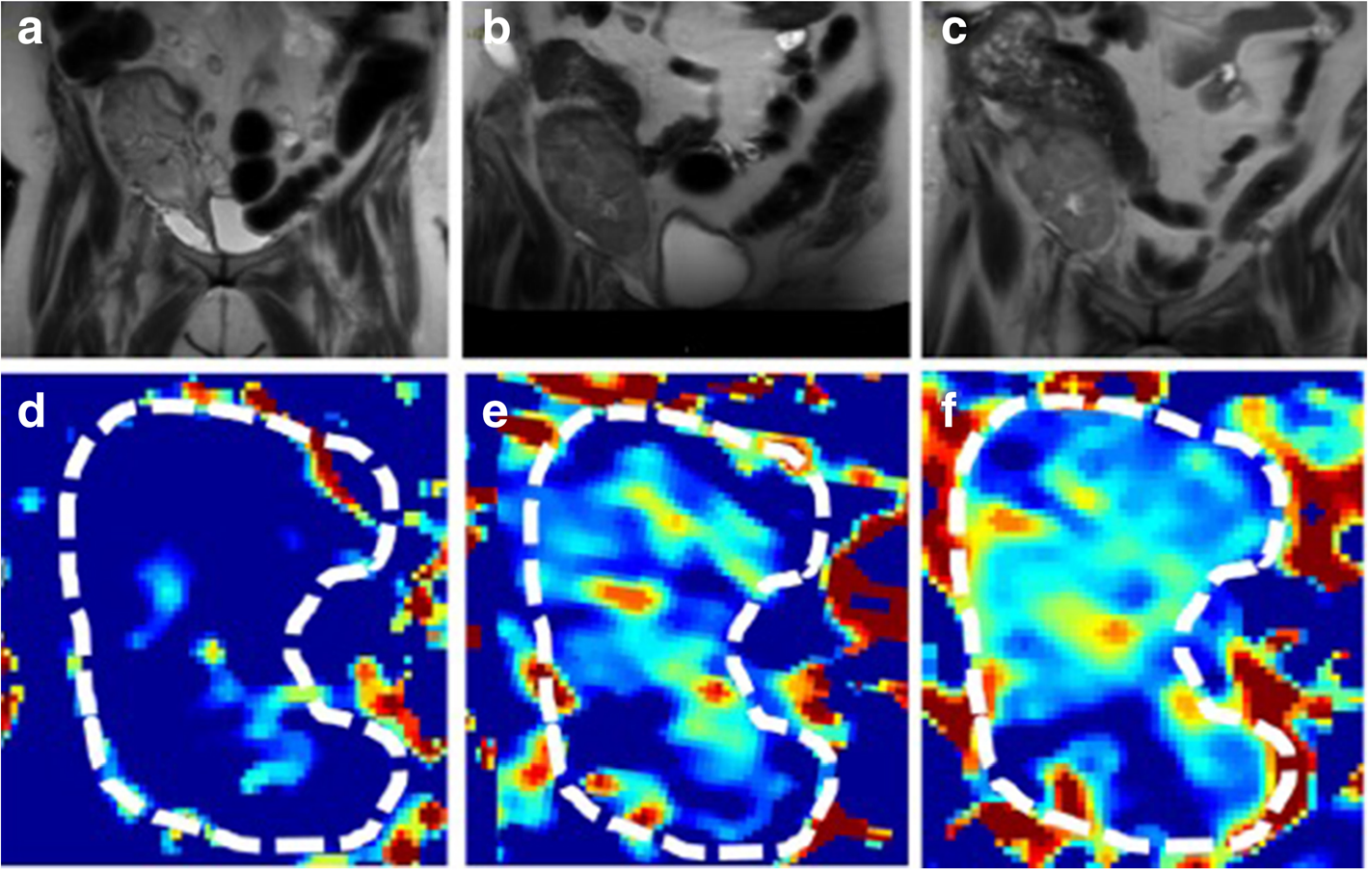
Serial diffusion-weighted MRI scans demonstrated improvements in allograft perfusion following transplant renal vein thrombectomy. (a – c) Serial conventional T2 weighted images, with grossly abnormal high T2 signal throughout the allograft parenchyma. (a) POD 22 scan. (b) POD 48 scan. (c) Scan at 3 months post-transplant vein thrombectomy. (d – e) Serial pseudocolorized perfusion (“f” fraction) maps derived from diffusion-weighted MRI images, progressing from (d) minimal perfusion on POD 22, to improved but still abnormally low perfusion (e) on POD 48 and (f) at 3 months post-thrombectomy. The *color bar* depicts the magnitude of the perfusion fraction, with *red* indicating higher perfusion, and *blue* indicating lower perfusion
